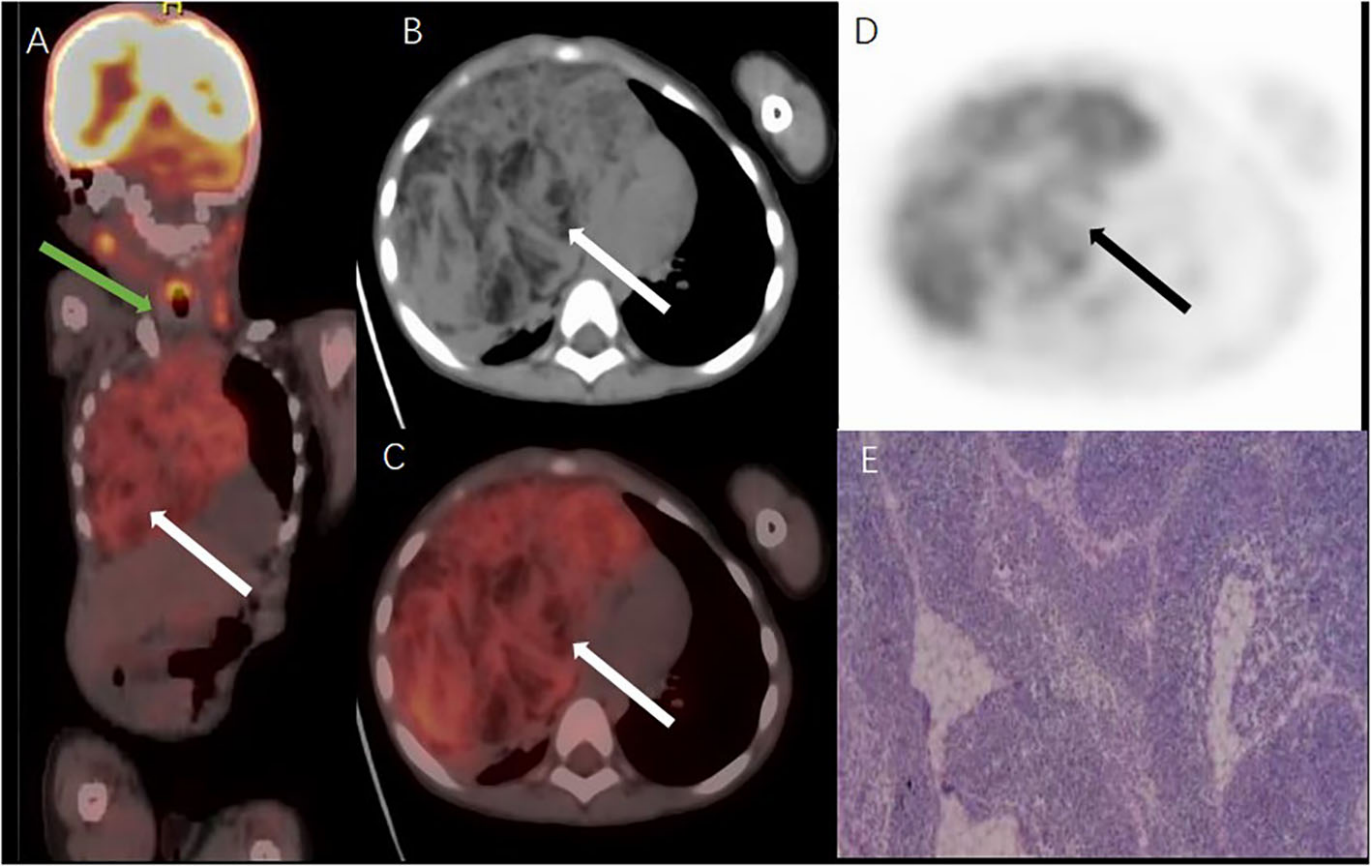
18F FDG PETCT images and pathologic picture.
